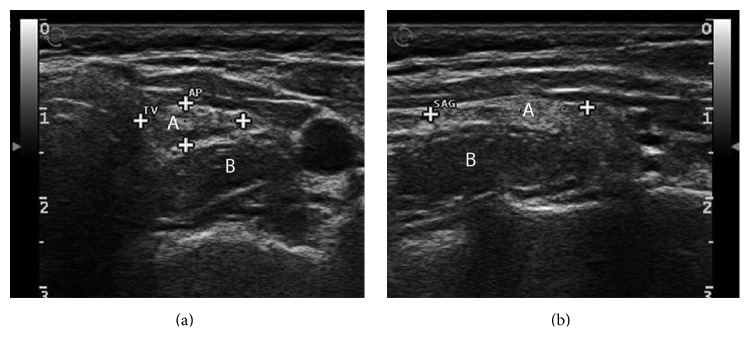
A: transverse (a) and longitudinal (b) ultrasound scans of the left thyroid lobe. B: transverse (a) and longitudinal (b) ultrasound scans of the esophageal lesion outside the posterior pole of the left thyroid lobe.
